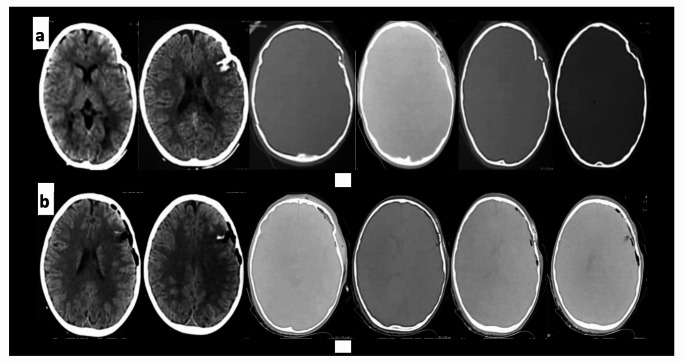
Young adult male patient with history of assault came to our ER with GCS 15/15, complaining from headache and vomiting. (a) Preoperative CT scans showing left fronto-parietal DSF with underling small cerebral contusion. (b) Postoperative CT showing good reconstruction of the skull defect using autologous bone fragments
